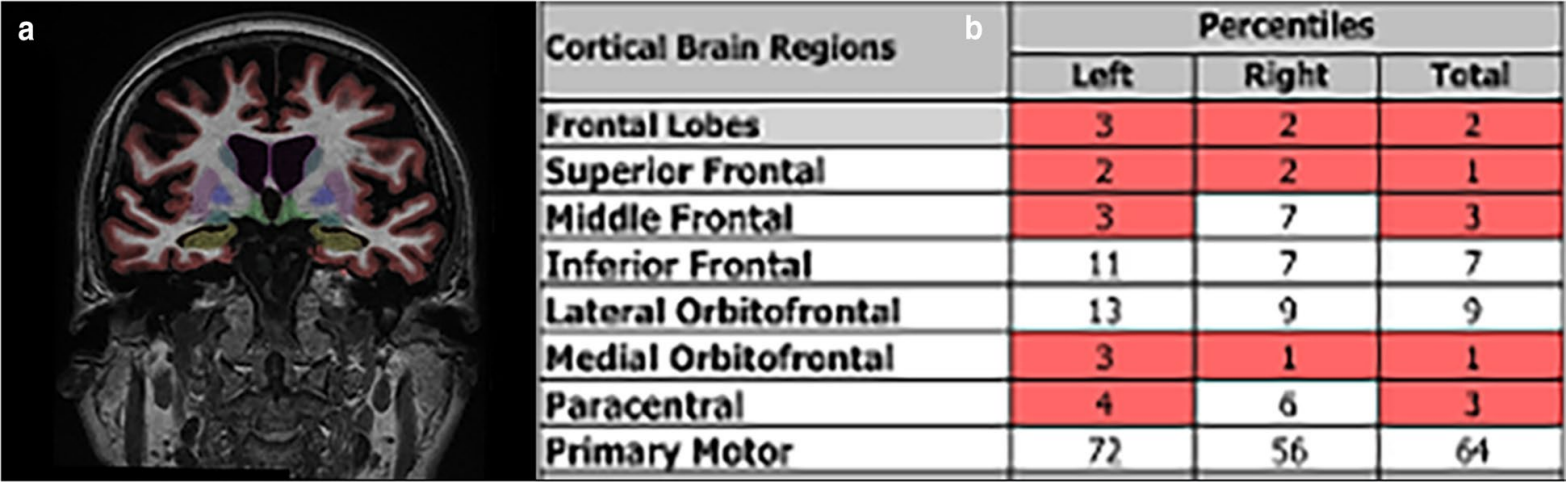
A 69-year-old female with odd behaviors including riding a children’s tricycle and impulsivity. Coronal volumetric T1-weighted images with proprietary automated segmentation software demonstrate volume loss in the frontal and temporal lobes (a). Volumetric quantitation identifies multiple regions in the frontal lobes in less than the fifth percentile of volume for age, including the medial orbital frontal lobes, typical of bvFTD (b)
